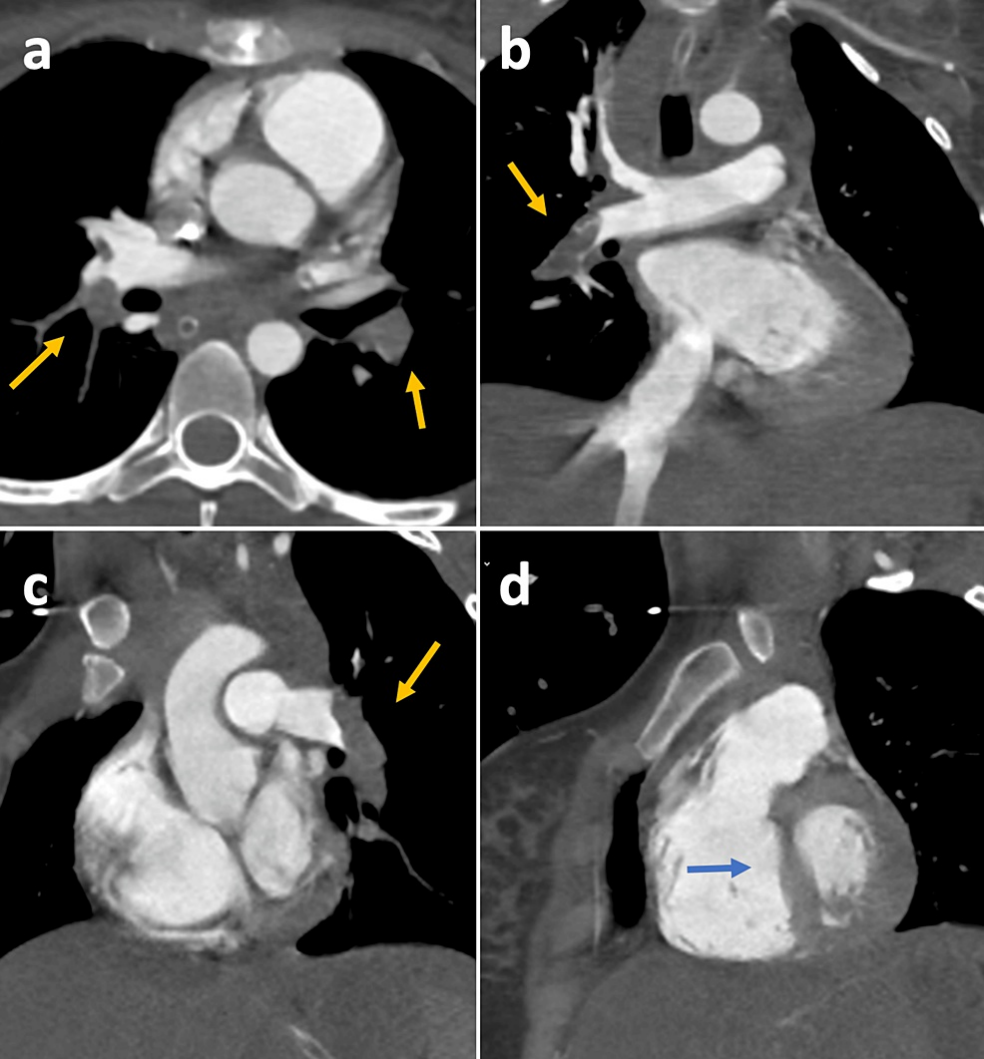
CT pulmonary angiogram with signs of acute PE Axial (a) and coronal oblique images (b,c) depicting multiple obstructive filling defects (yellow arrows); coronal oblique image (d) showing indirect signs of pulmonary hypertension, namely, right ventricular dilatation and interventricular septum flattening (blue arrow).
